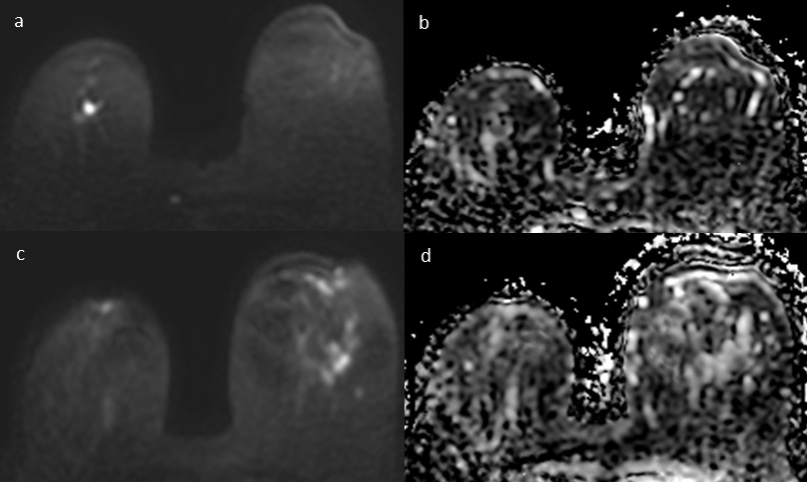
54-year-old female with bilateral breast cancer, invasive ductal carcinoma on the right breast and ductal carcinoma *in situ* on the left breast. (a) Axial DWI image (b value = 1000 s/ mm^2^) shows an hyperintense lesion between the upper quadrants of the right breast, (b) with corresponding 0,8 × 10^−3^ mm^2^/s ADC values. (c) Axial DWI image (b value = 1000 s/mm^2^) shows an hyperintense area in the upper outer quadrant of the left breast, (d) with higher ADC values (1,02 × 10^−3^ mm^2^/s). ADC, apparent diffusion coefficient; DWI, diffusion-weighted imaging.
